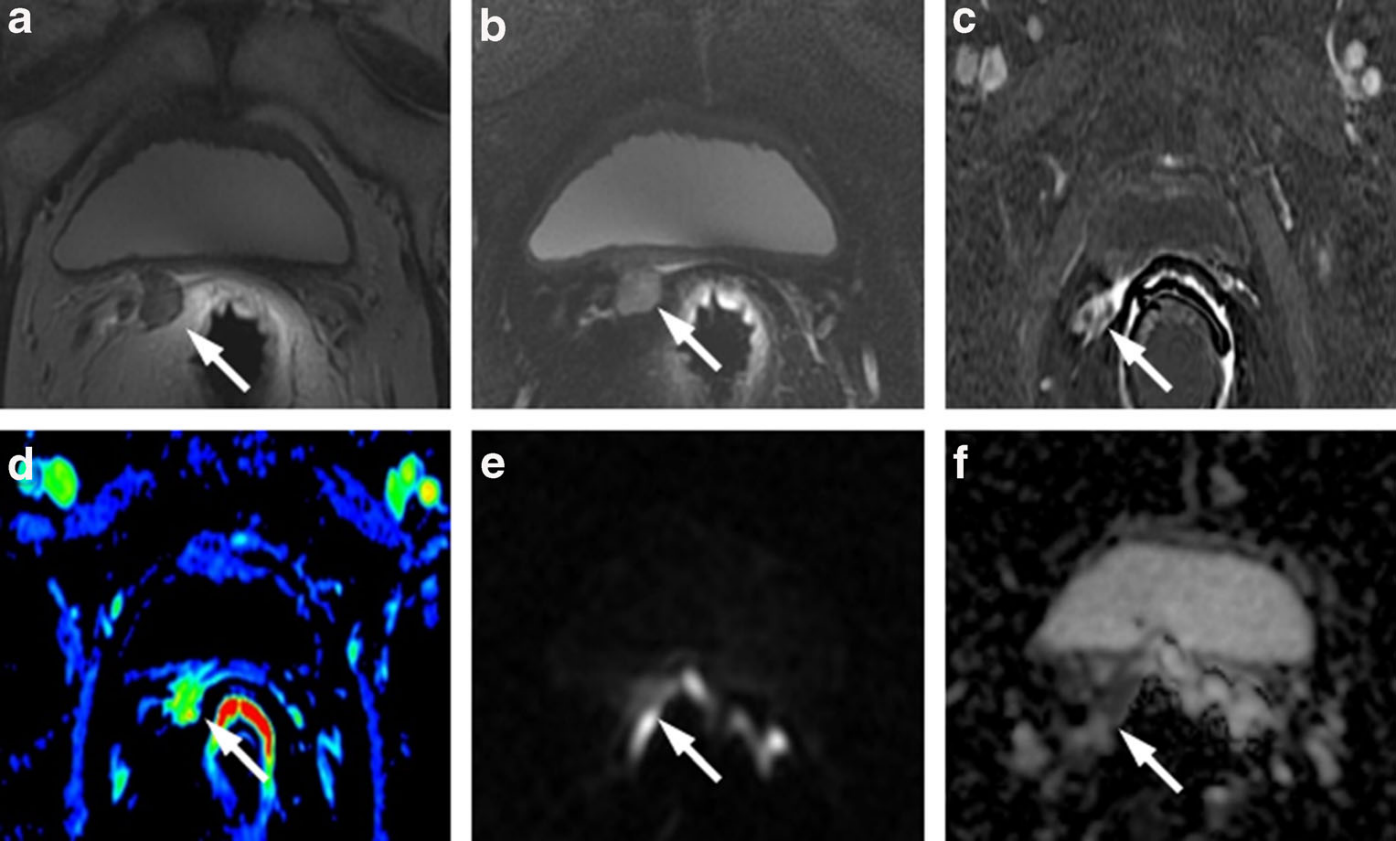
MR images of a 68-year-old man with prostate-specific antigen progression (PSA serum level 0.67 ng/mL) after radical retropubic prostatectomy, with suspected local recurrence. a Axial T2-weighted fast spin-echo (6567/111) and b axial T2-weighted fast spin-echo fat saturated (7216/118) show on the zone previously occupied by the proximal portion of right seminal vesicle, a hyperintense nodular tissue (arrow). c Gradient-echo T1-weighted subtracted image and d colour DCE map shows a well-defined area of marked enhancement (*arrow*) on the same location as the solid nodular tissue seen on T2-weighted images. e Diffusion weighted images with a b value of 3.000 s/mm^2^ and f ADC map show a focal area of restricted diffusion (*arrow*) corresponding to the solid nodular tissue detected on T2-weighted images and the enhancing zone seen on dynamic contrast-enhancing images. These findings are consistent with local recurrence (*white arrows*)
